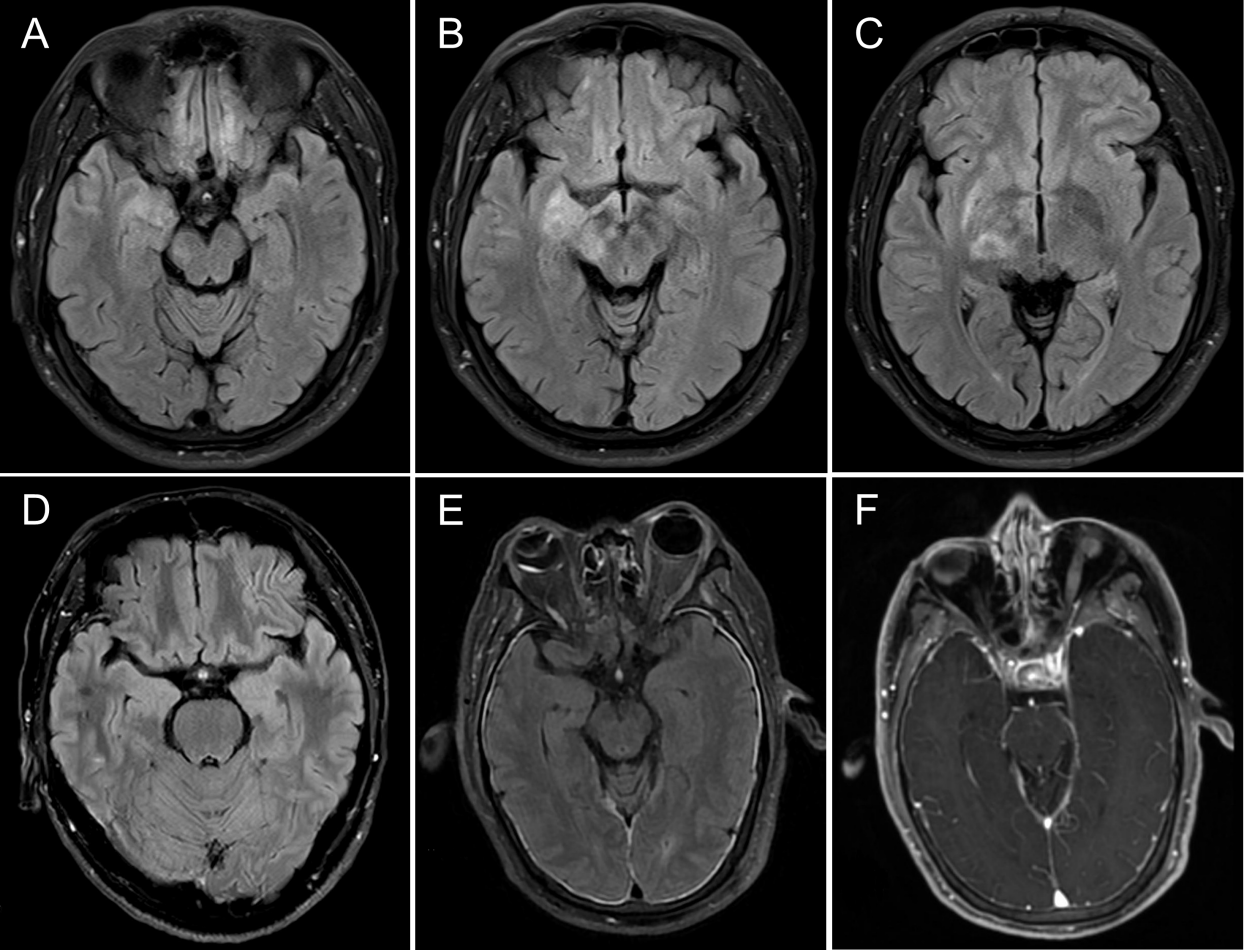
MRI of representative patients with anti-mGluR5 encephalitis in the current study. (A-C) Brain MRI of patient 2. Initial brain MRI at disease onset showed T2/fluid-attenuated inversion recovery hyperintensities of the right mesiotemporal lobe (A), cerebral peduncle (B), thalamus, and putamen (C). (D) Brain MRI of patient 1. Brain MRI at disease onset and at the first relapse was normal, but at the second relapse, brain MRI showed T2/fluid-attenuated inversion recovery hyperintensity of the bilateral hippocampi. (E, F) Brain MRI of patient 5. Initial brain MRI at disease onset showed diffuse T2/fluid-attenuated inversion recovery hyperintensity of the meninges and enhanced on post-gadolinium T1-weighted images.
